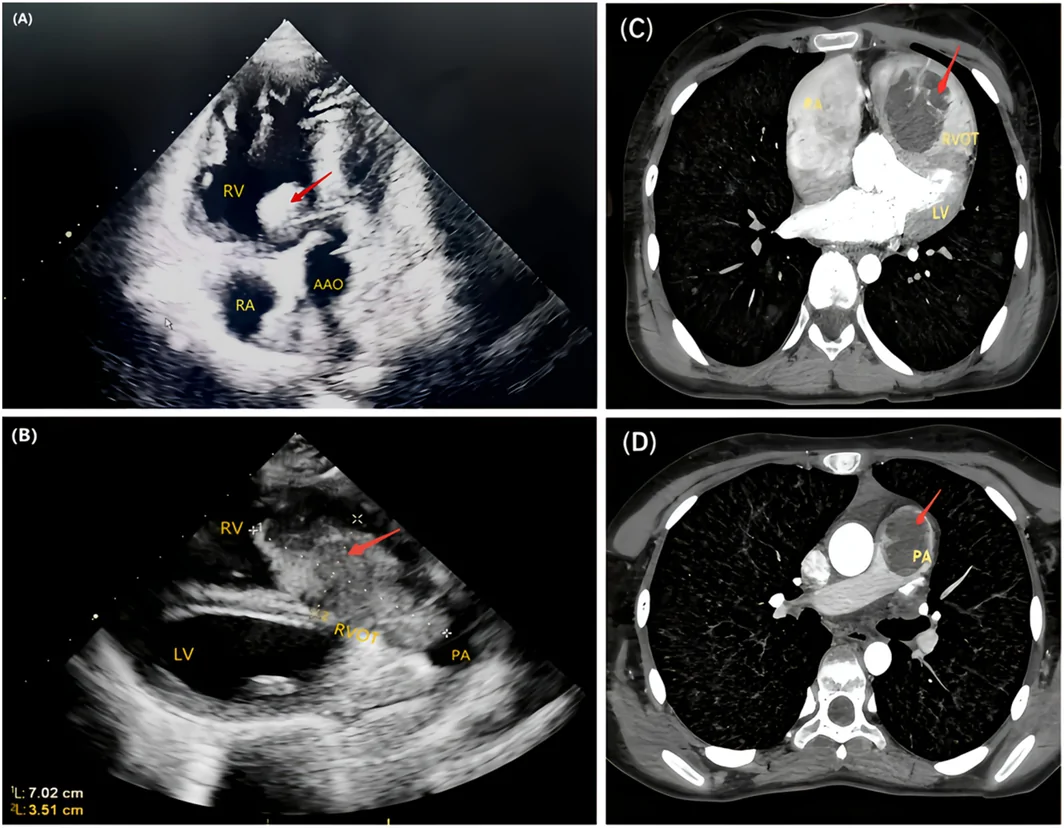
Preoperative imaging findings. (A–B) Transthoracic echocardiography images: (A) A mass in the RV (arrow). (B) The mass extending into the RVOT and PA (arrow). (C–D) Computed tomography images: (C) A mass in the RVOT (arrow). (D) A mass in the PA (arrow). AAO, ascending aorta; LV, left ventricle; PA, pulmonary artery; RA, right atrium; RV, right ventricle; RVOT, right ventricular outflow tract.
